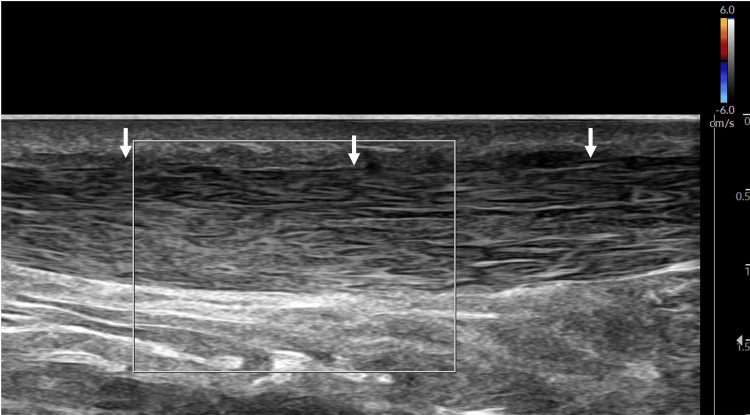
Long-axis view of the Achilles tendon with the color Doppler tool showing no hyperemia within the tendon. Arrows: Achilles tendon.
